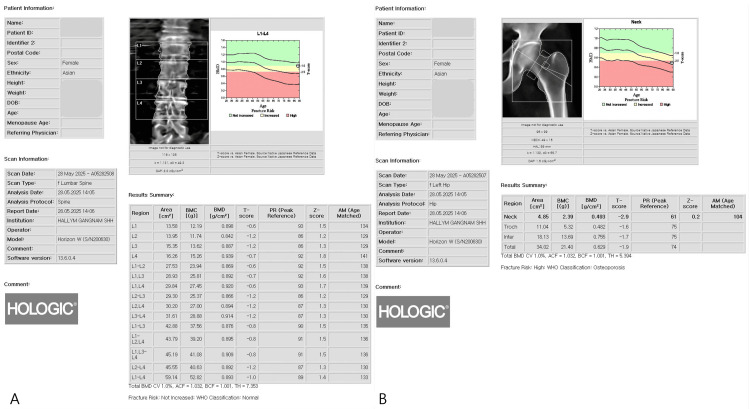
DXA assessment of BMD (A) Lumbar spine measurements showing BMD, BMC, and T-scores at each vertebral level. (B) Hip measurements demonstrating region-specific BMD, BMC, and T-scores DXA: dual-energy X-ray absorptiometry; BMD: bone mineral density; BMC: bone mineral content
